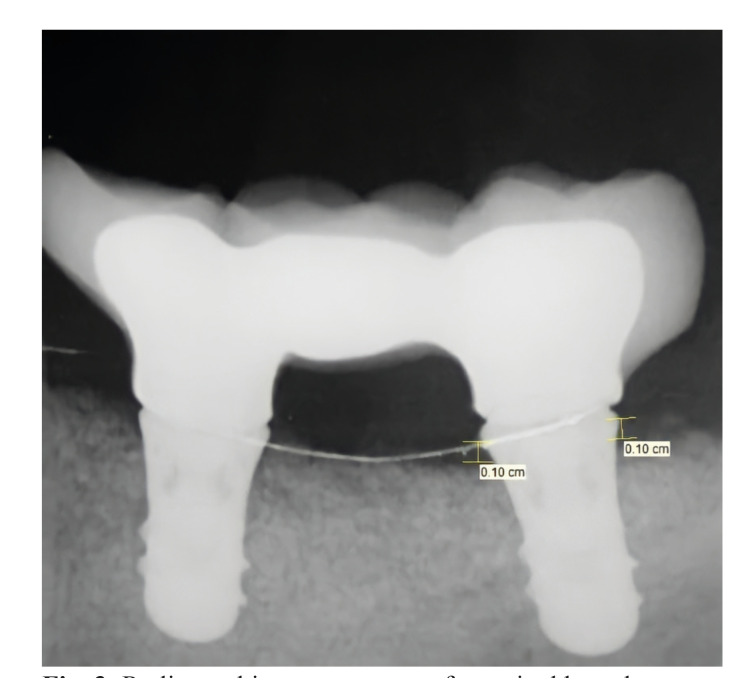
Radiographic measurement of marginal bone loss.
